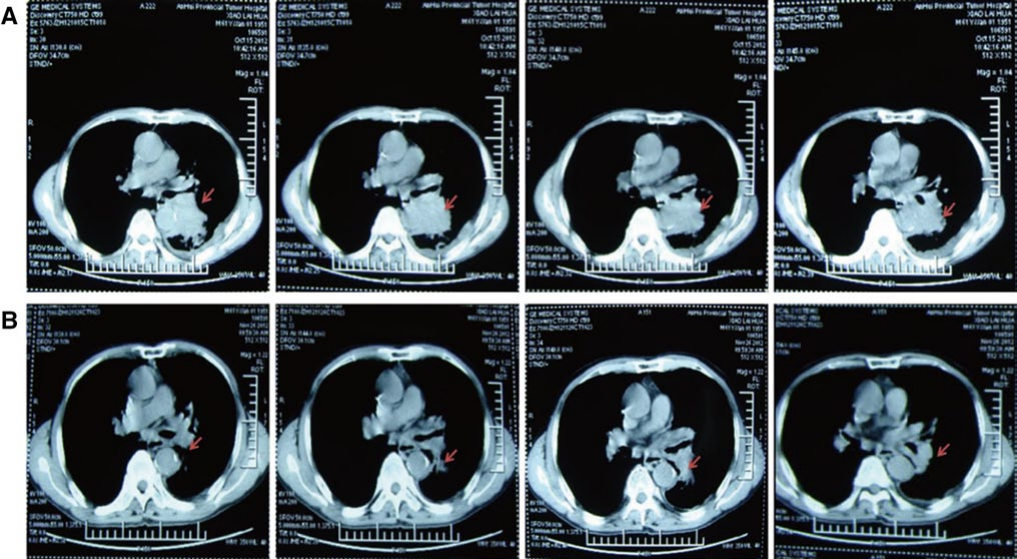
CT scan of the patient with moderately differentiated squamous cell carcinoma of the *left* lung complicated by *right* supraclavicular lymph node metastases before (a) and after (b) interventional therapy. The *arrows* showed the position of lesions
